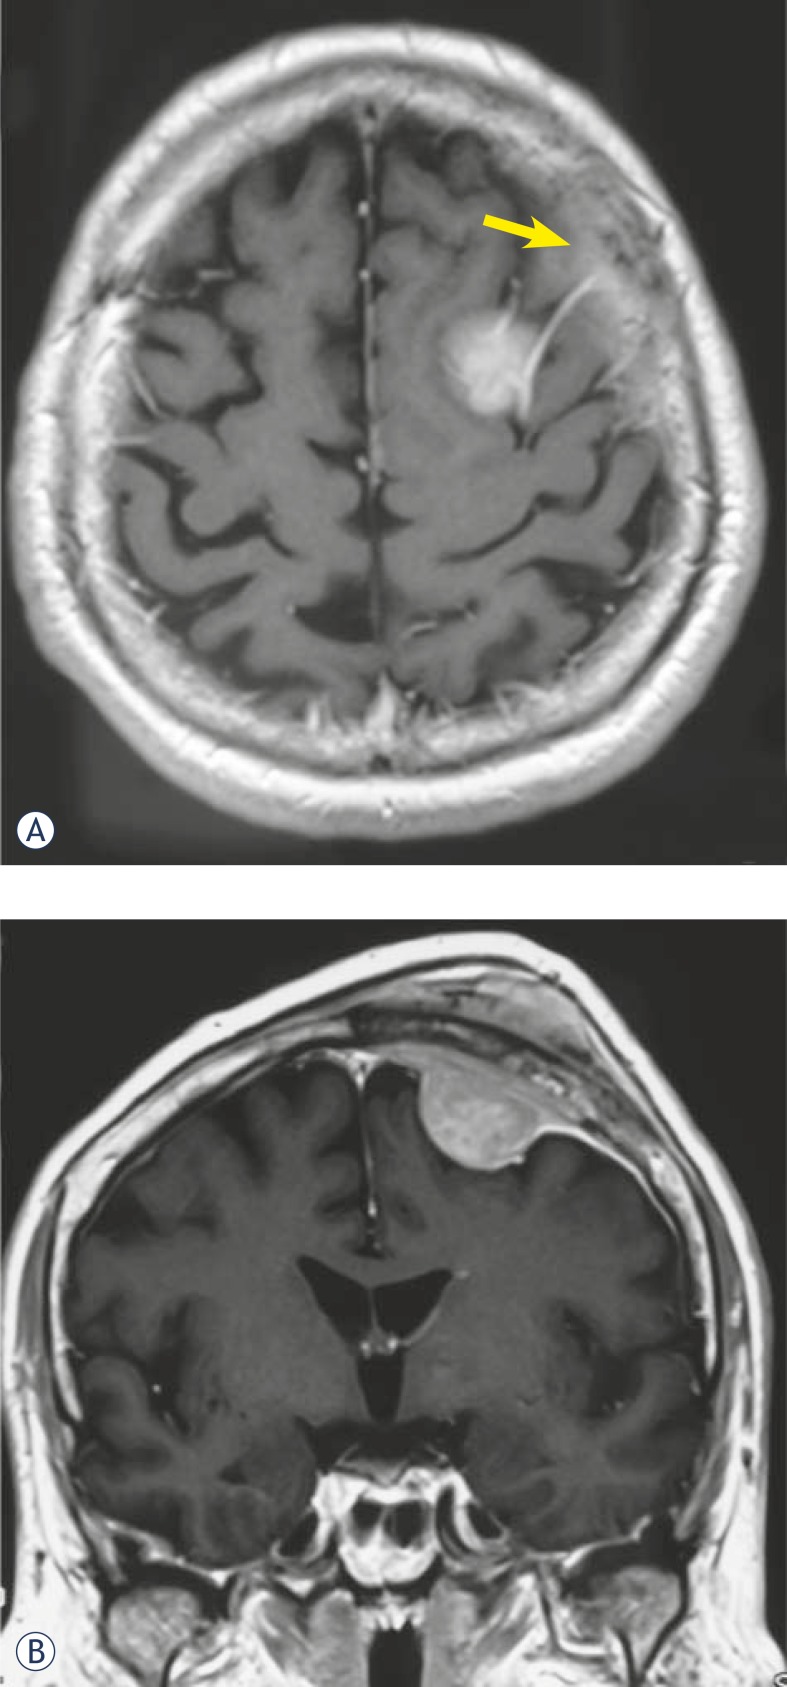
The axial view of the CT scan showing homogenous lesion in the motor/premotor area, radiologically classified as meningioma, with hyperostotic bone (arrow) (A). The coronal view of the tumour, growing intra- and extracranially (B).
